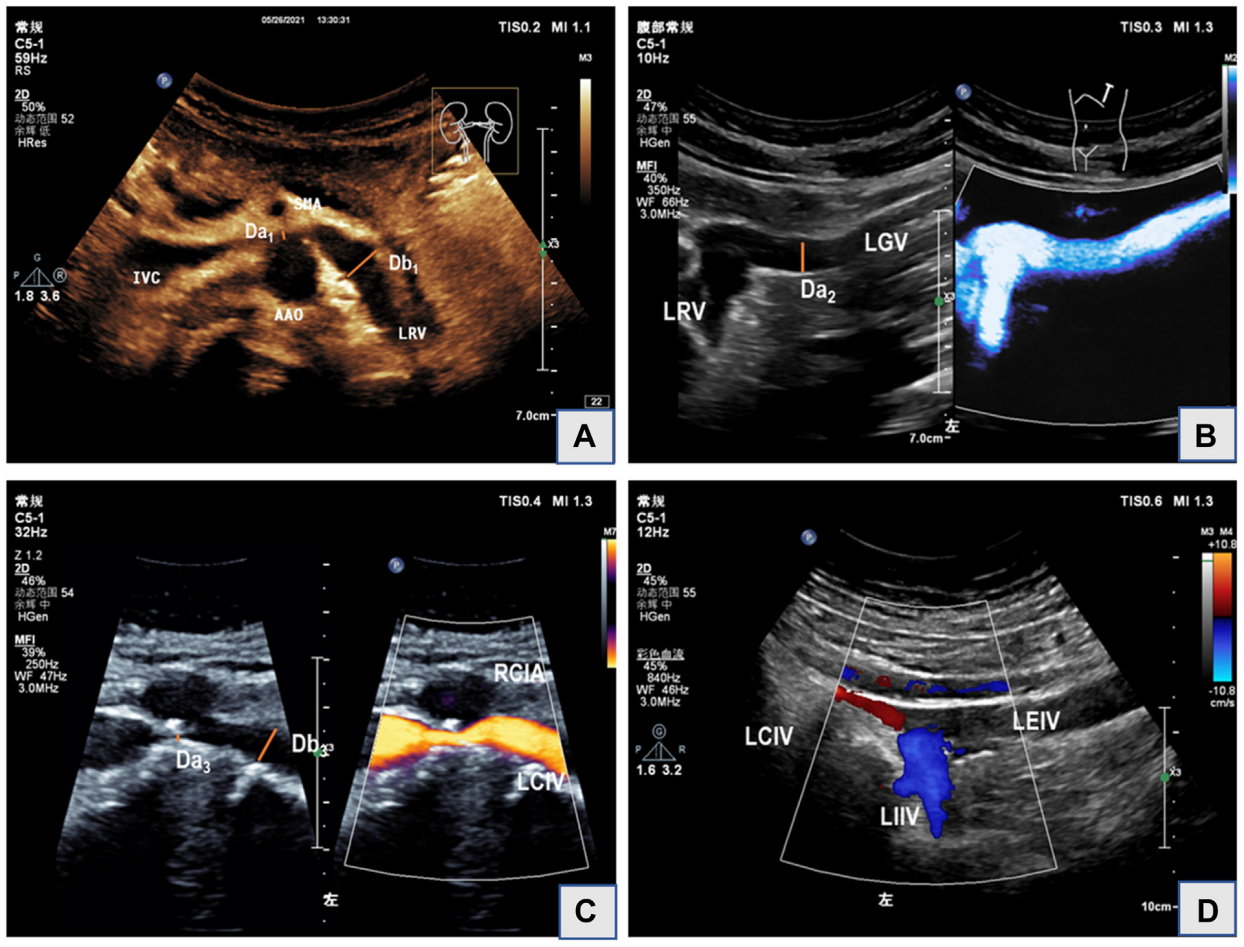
Ultrasound images of the abdomino-pelvic vessels. A, Nutcracker phenomenon, in which the left renal vein (*LRV*) is compressed at the space between the superior mesenteric artery (*SMA*) and abdominal aorta. (*Da1*, narrowest point at where the LRV crosses the angle between the SMA and abdominal aorta (*AAO*). *Db1*, the point at which the LRV dilation is the most pronounced.). B, Left ovarian vein (*LOV*) reflux. Color Doppler imaging demonstrates reversed flow within the LRV and LOV. (*Da2*, maximum diameter of LOV/left gonadal vein [*LGV*]). C, Left common iliac vein (*LCIV*) compression. A longitudinal view of the LCIV imaged in B-mode and color Doppler imaging shows the compression of the LCIV by the right common iliac artery (CIA) on top and vertebrae underneath. (*Da3*, the luminal diameter of the LCIV at the point where the right CIA crosses the LCIV. Db3, the distal section of the LCIV). D, Internal iliac vein (IIV) reflux. Color Doppler imaging shows reversed flow within the left IIV. *LEIV*, Left external iliac vein; *LIIV*, left internal iliac vein; *IVC*, inferior vena cava; *RCIA*, right common iliac artery.
